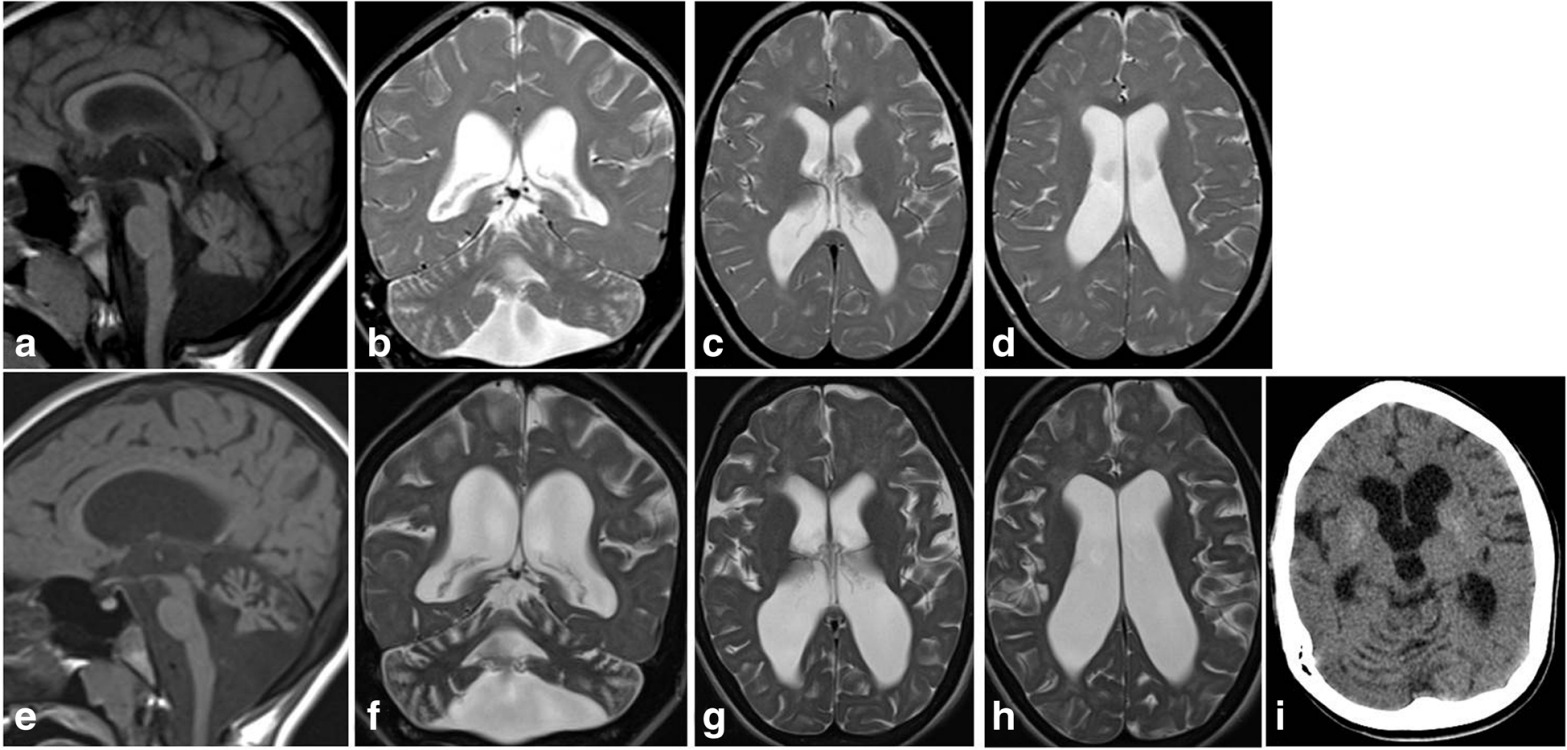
Cockayne syndrome –Brain MRI at age 5 years, top (a-d), brain MRI at age 9 years, bottom (e-h), and brain CT at age 9, (i). Note the diffuse cerebral atrophy progression, mainly in the posterior fossa, and the diffuse slight white matter hyperintensity due to hypomyelinating leukoencephalopathy. CT demonstrate faint hyperdensity in the putamina due to fine calcification
